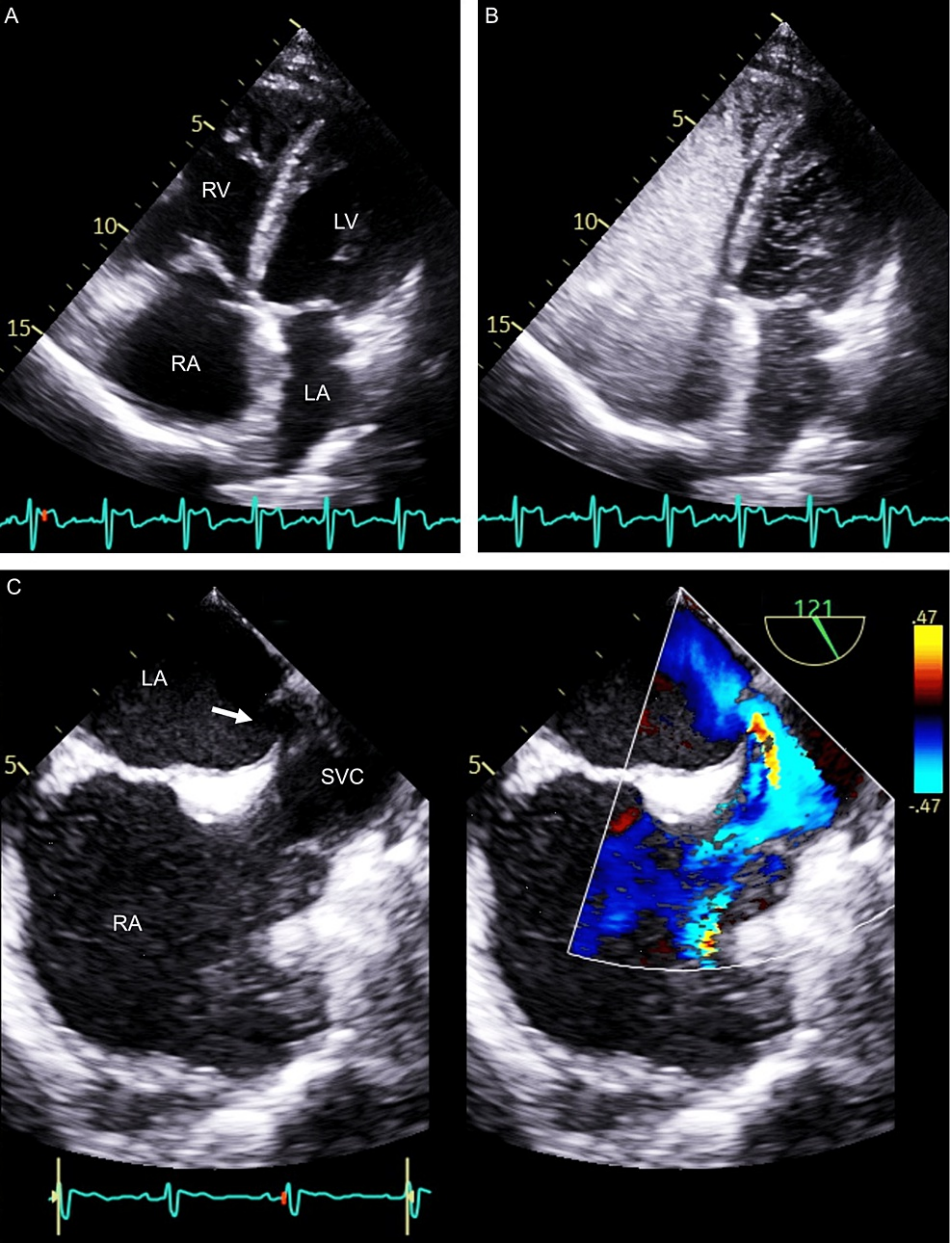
Echocardiography Transthoracic echocardiographic four-chamber images before (A) and after agitated saline contrast (B) show not only contrast-enhanced right atrium (RA) and right ventricle (RV) but also numerous bubbles in the left atrium (LA) and left ventricle (LV). Transesophageal echocardiography (C) shows a wall defect between the superior vena cava (SVC) and LA (arrow) with direct blood flow from LA to SVC on color Doppler.
